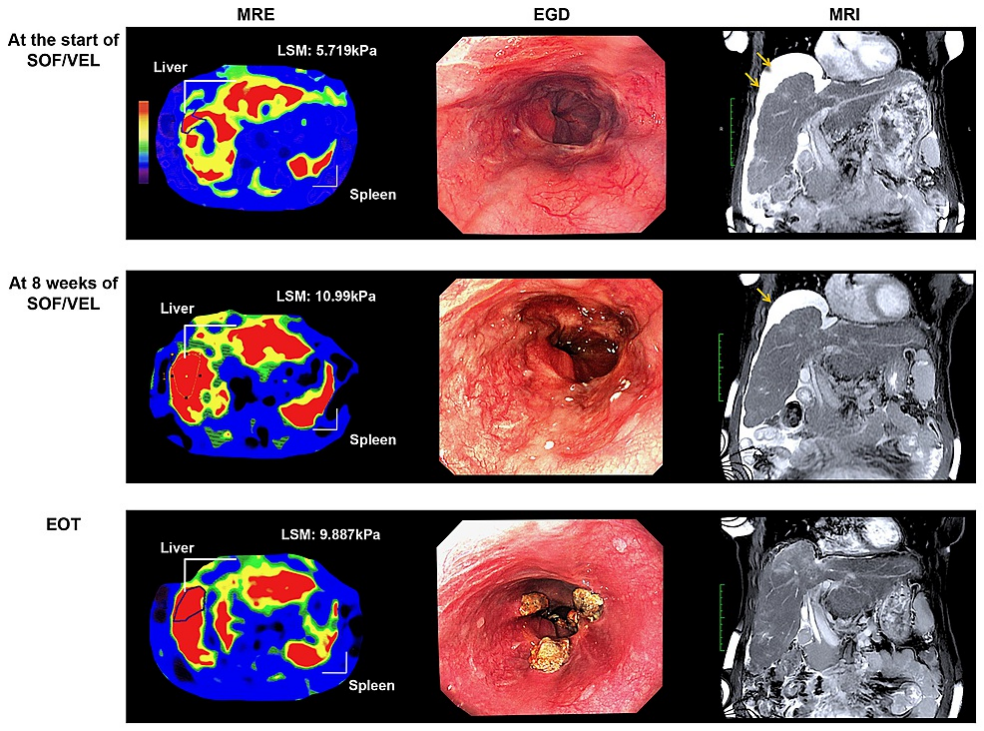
MRI and EGD images before, during, and at the end of treatment Images of liver and esophageal varices before SOF/VEL therapy (top row), after eight weeks of therapy (middle row), and at the end of therapy (bottom row). From left to right: MRE, EGD, and MRI of coronal sections. EGD images at the end of therapy are after re-treatment of esophageal varices. MRE shows the color mapping of the shear stiffness (kPa) of the organ, which changes from blue to red as the stiffness increases. In the present case, liver stiffness increased markedly to 10.99 kPa at eight weeks of treatment. The color mapping of the spleen also suggested an increase in stiffness. SOF, sofosbuvir; VEL, velpatasvir; MRE, magnetic resonance elastography; EGD, esophagogastroduodenoscopy; MRI, magnetic resonance imaging
